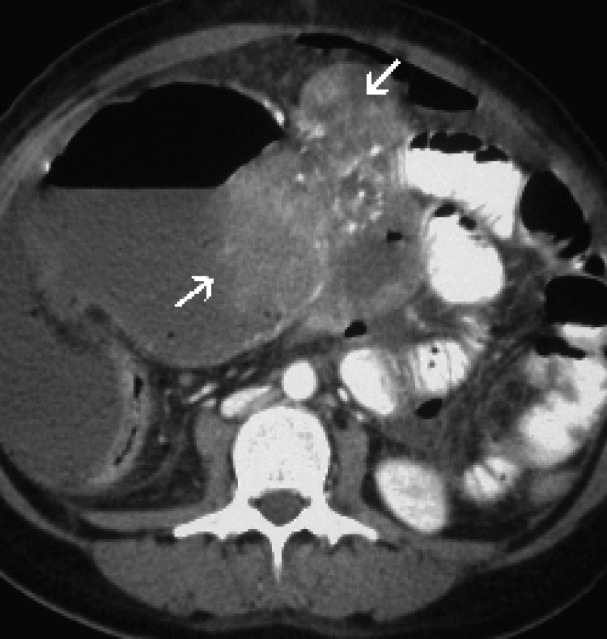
A postcontrast computed tomographic scan showed a huge primary calcified GIST (arrows) with a cystic component.
